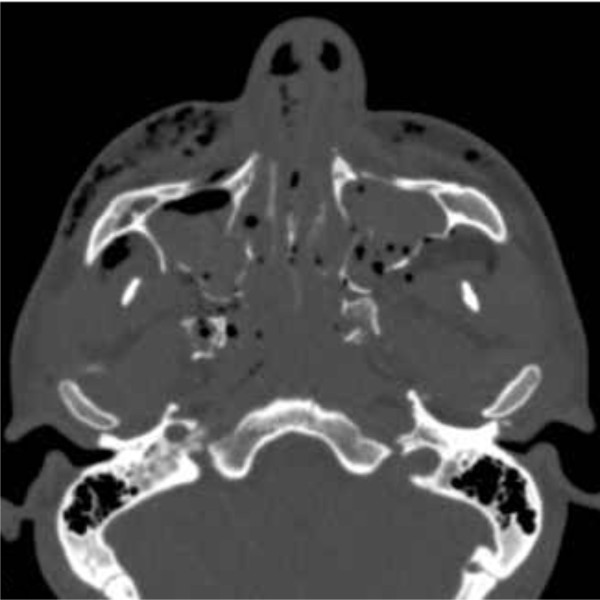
Pre-operative computed tomography scan in axial projection demonstrating bilateral pterygoid process fracture.
